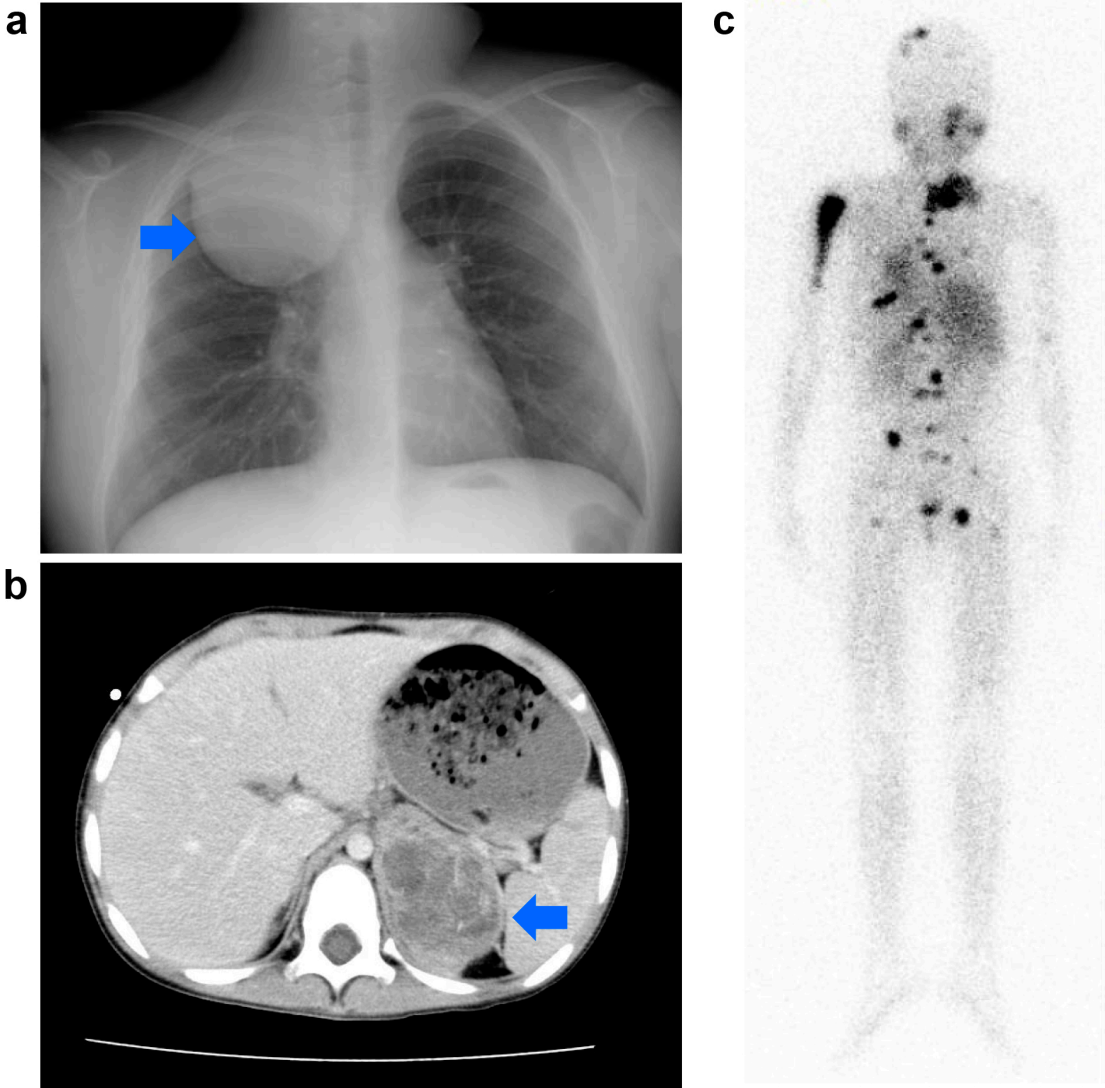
Clinical presentation of neuroblastoma. (a) Localized thoracic neuroblastoma. The arrow indicates tumor mass. (b) Enhanced abdominal computed tomography. The arrow indicates a large tumor in the adrenal medulla. (c), Metastatic neuroblastoma revealed by metaiodobenzylguanidine (MIBG) scintigraphy.
